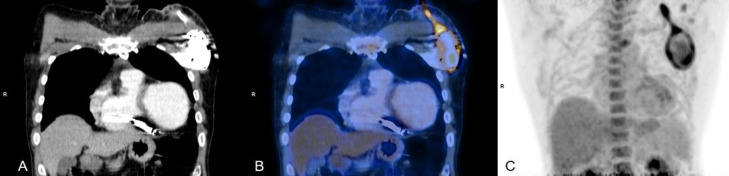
Coronal section (A) CT , (B) fused FDG PET CT and (C) Maximum Intensity Projection (MIP) images showing abnormal increased FDG uptake (SUV_Max_: 6.7) around the pacing box extending to the proximal lead wire
